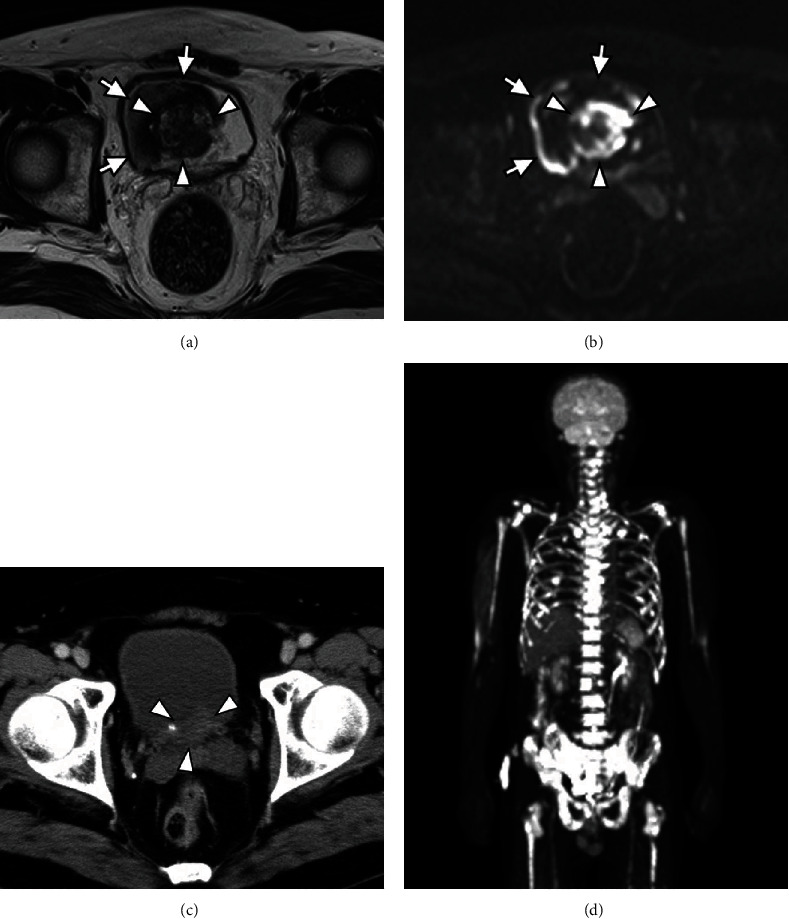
(a) MRI T2-weighted image and (b) diffusion-weighted image showed a bladder tumor (white arrowheads) with hematoma (white arrows). (c) The CT image after NAC did not show rapid progression of the bladder tumor (white arrowheads). (d) The FDG-PET findings when the patient presented with low back pain after the RC.
